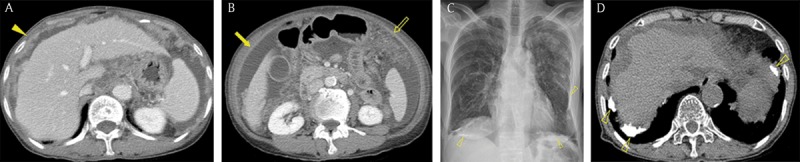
An 80-year-old woman with peritoneal malignant mesothelioma who had a history of asbestos exposure for more than 20 years. (A, B) Axial portal venous phase abdominal CT images showed multiple nodules (arrowhead), peritoneal thickening (arrow), ascites, and omental haziness (open arrow), findings that mimicked peritoneal carcinomatosis. (C) Chest radiograph demonstrated pleural opacities (open arrowheads) in the bilateral hemithorax. (D) An axial unenhanced chest CT image demonstrated multiple calcified plaques (open arrowheads) in both pleura.
